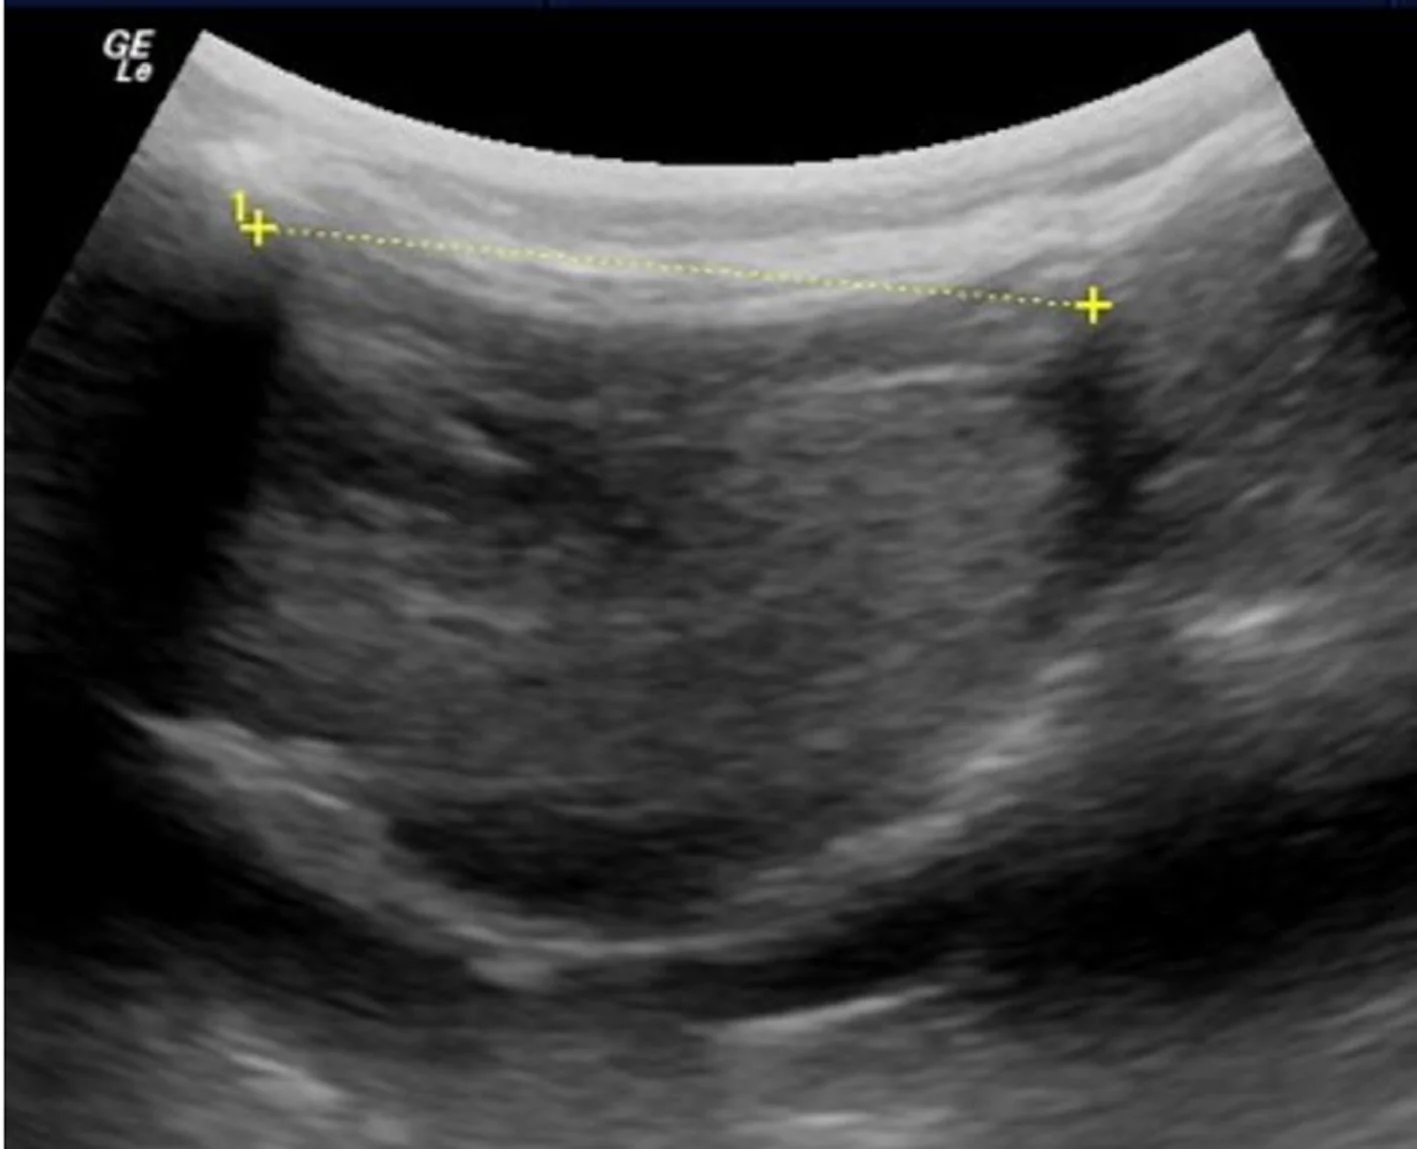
Airway ultrasound in children with measurement of the hyo-mental distance (HMD). For this measurement, the probe is placed in the sagittal plane in the submental area.
